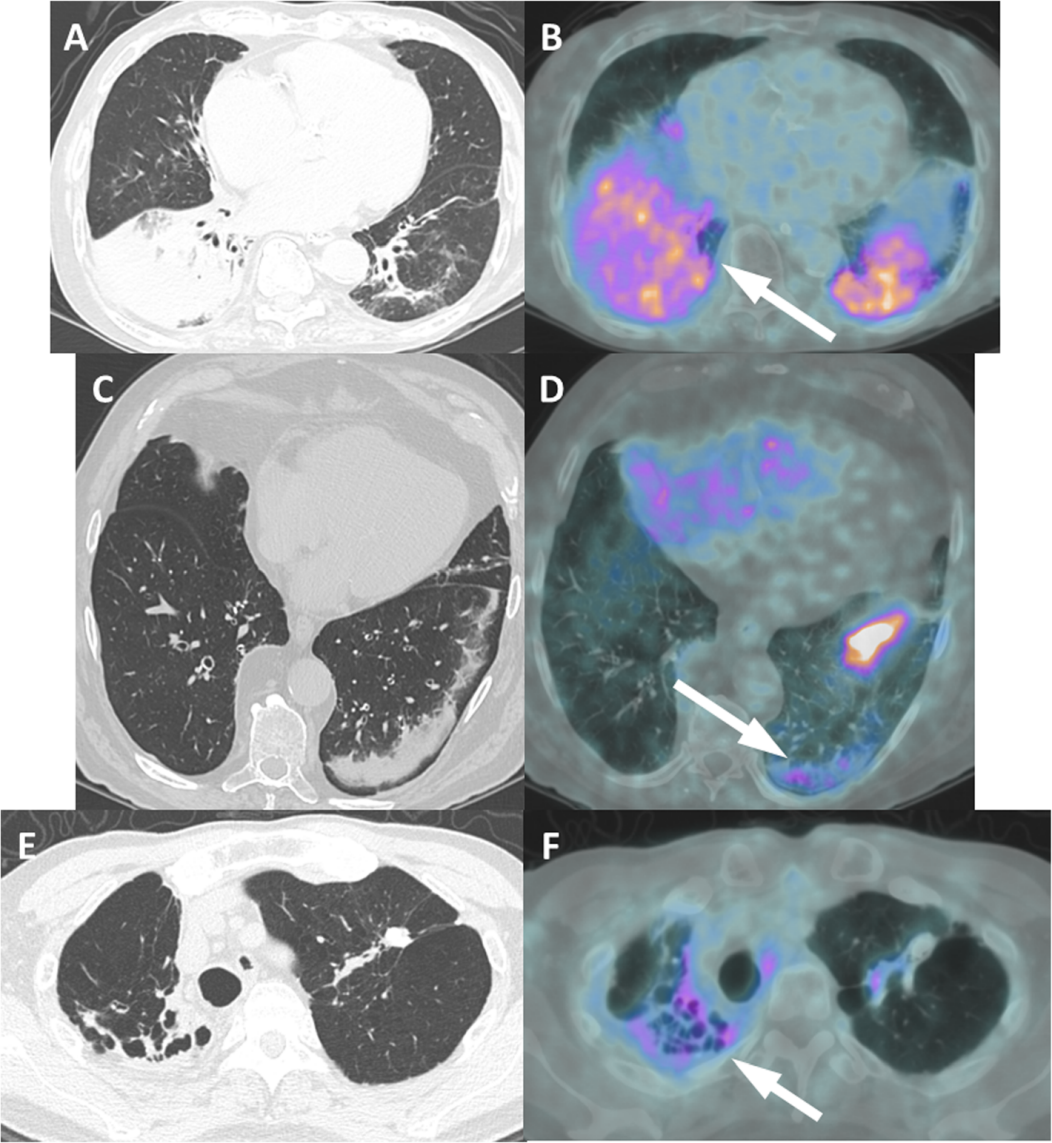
Pulmonary diseases with PSMA uptake. Patient 1, pulmonary consolidation (a, b). Axial CT (a) and PET/CT (b) show a pulmonary consolidation with air bronchogram in both lower lobes (arrow on B) of the lungs in a 90-year-old patient presenting cough and fever. Patient 2, pulmonary atelectasis (c, d). Axial CT (c) and PET/CT (d) show a laminar atelectasis in both lower lobes of the lungs with tiny PSMA uptake (arrow on D). Patient 3, tuberculosis sequelae (e, f). Axial CT (e) and PET/CT (f) show retractile bronchiectasis in the upper bilateral lung lobes with a few calcifications and mild uptake (arrow on F), suggestive of tuberculosis sequelae
